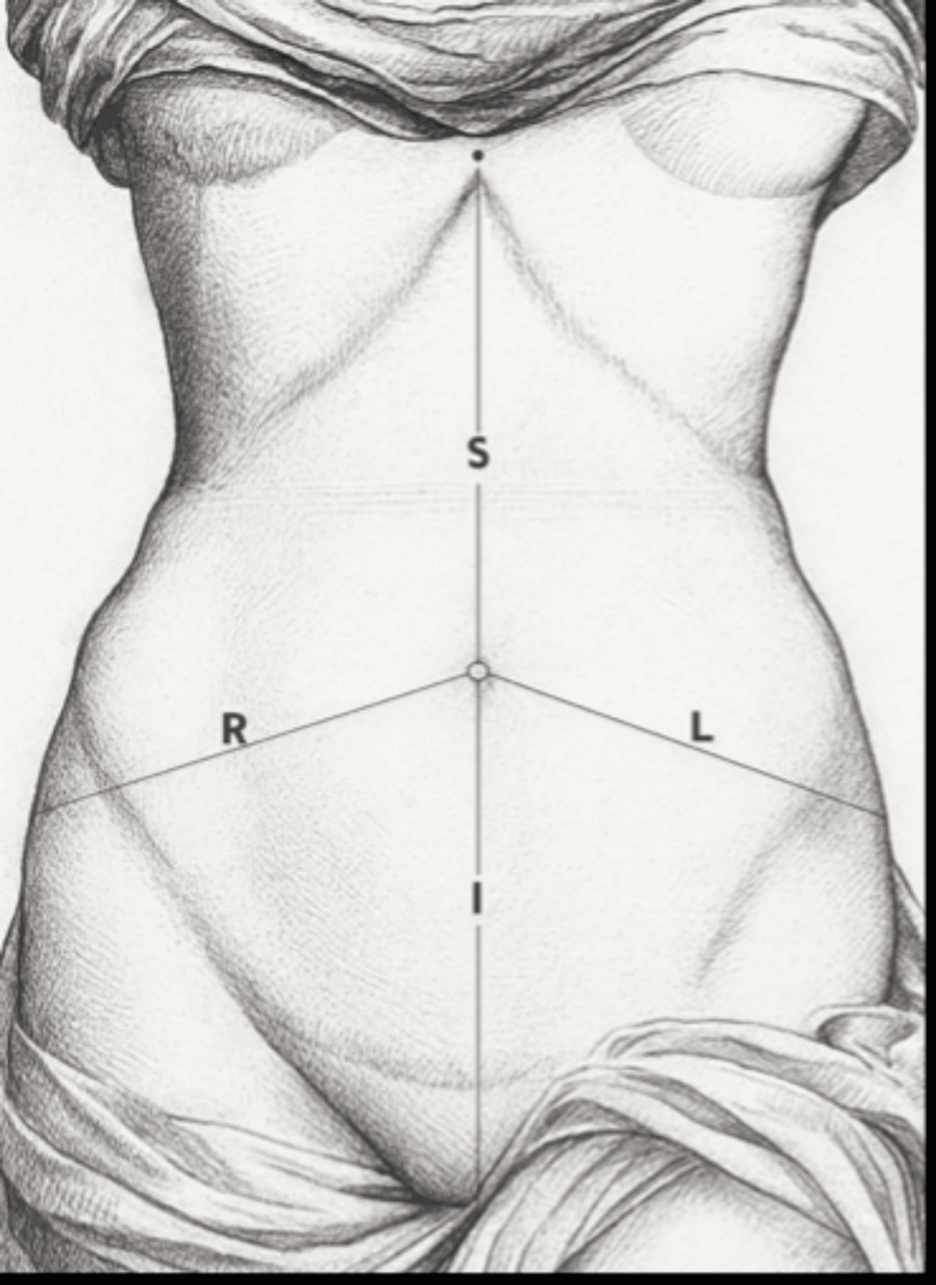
Markings of the points for ultrasonographic measurements S: midpoint of the xiphisternum and umbilicus. I: midpoint of the umbilicus and pubic symphysis. R (right), L (left): midpoint of the anterior superior iliac spine (ASIS) and umbilicus. Image Credit: Authors' original creation.
